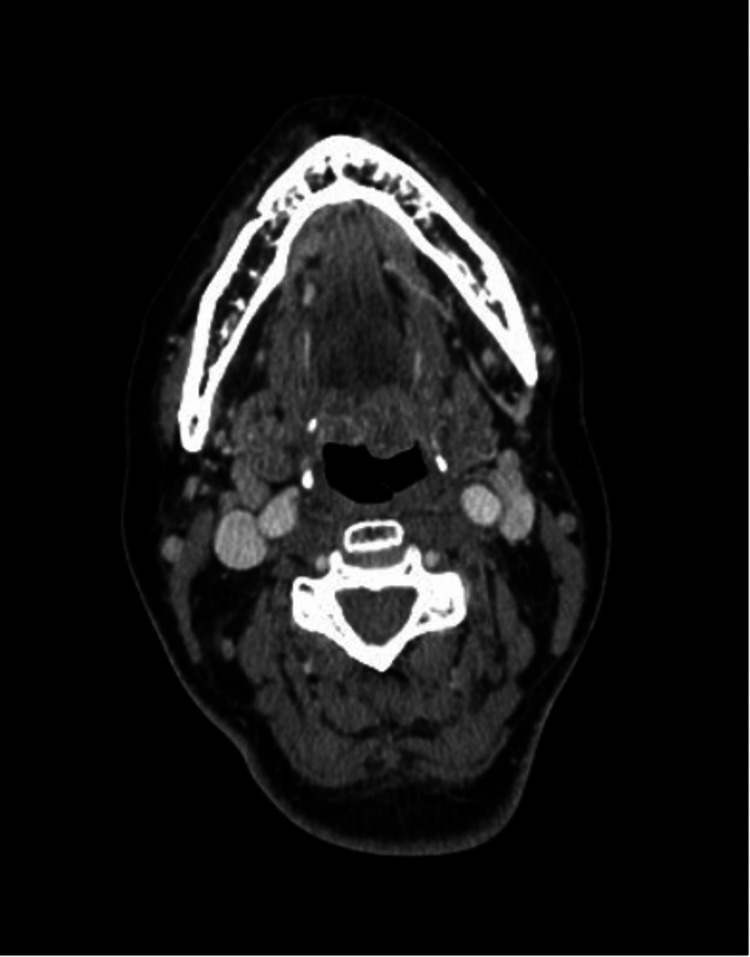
Computed tomography showing no enhancing lesions consistent with complete response.
